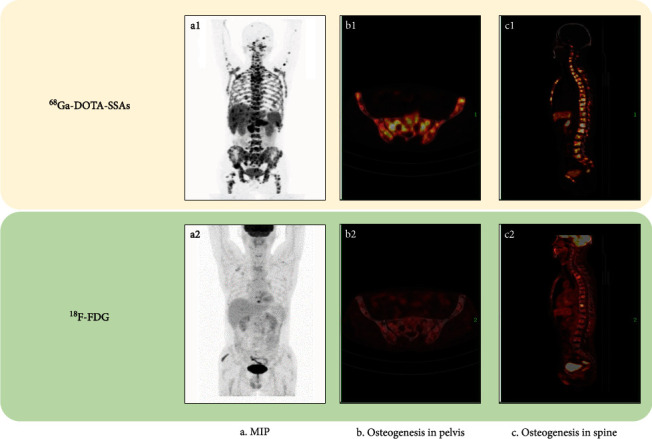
Dual-tracer imaging in a 57-year-old man with rectal NET G2 (Ki-67 index 3%), accompanied by liver, lymph node, and bone metastases. The patient suffered from systemic diffuse bone metastases, including in the spine, pelvis, ribs, sternum-clavicle-scapula, proximal limbs, and skull. Computed tomography findings mainly manifested osteogenesis changes. b1 and c1 show more bone lesions than ^18^F-FDG PET/CT imaging. (a–c) Maximum intensity projection, osteogenesis in the pelvis, and osteogenesis in the spine, respectively, detected by dual-tracer imaging.
